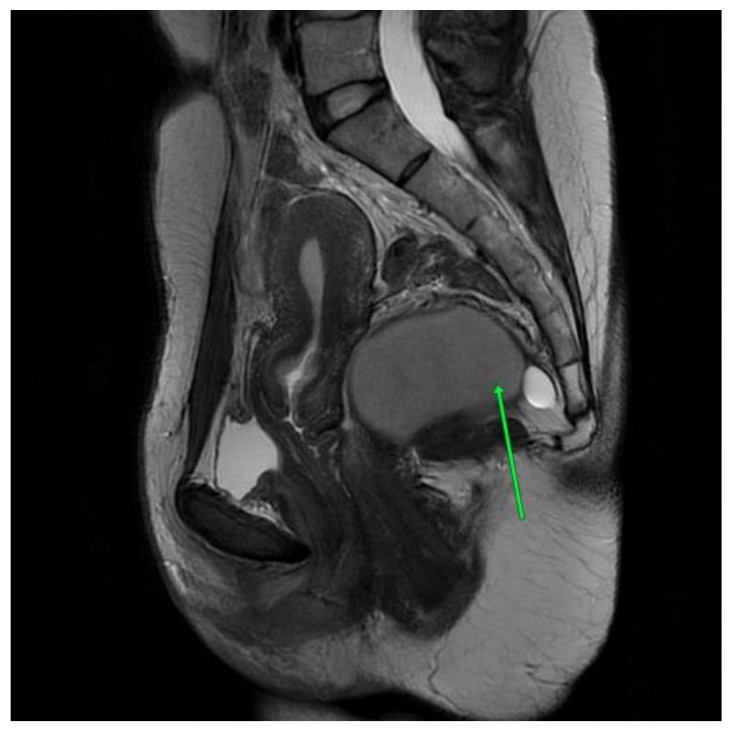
First patient MRI scan of the lesser pelvis. Green arrow shows the lesion.
